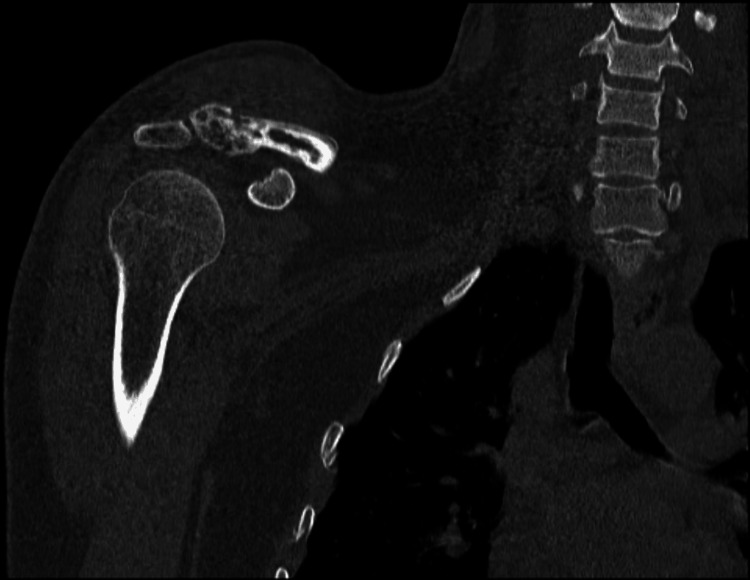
Preoperative CT scan revealing a significant osteolytic lesion at the distal clavicle with an accompanying soft tissue component.
